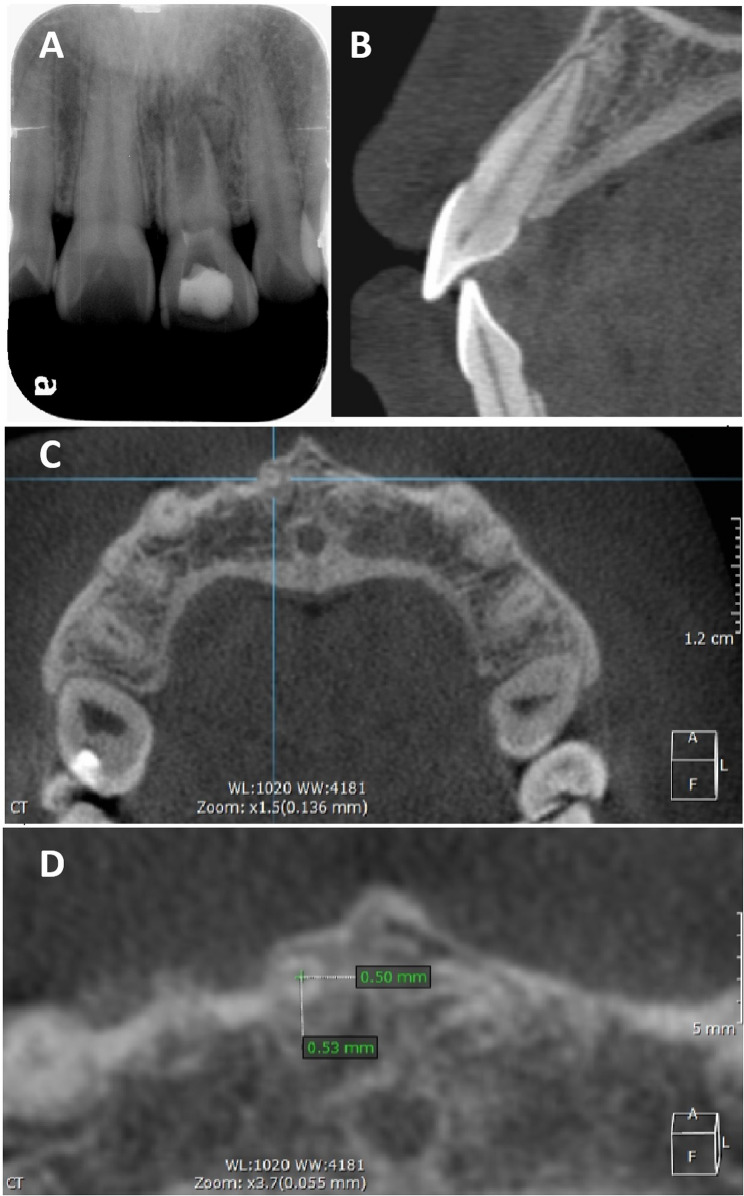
Representative case of tooth 11. The patient was 16 years old, and the tooth was classified as mature with apical closure. A shows the periapical radiograph, (B) a sagittal CBCT image view of the tooth, (C) an axial CBCT image view of the apical foramen and (D) a magnified axial CBCT image view showing measurement of the apical constrictor diameter in the mesiodistal and faciolingual directions
